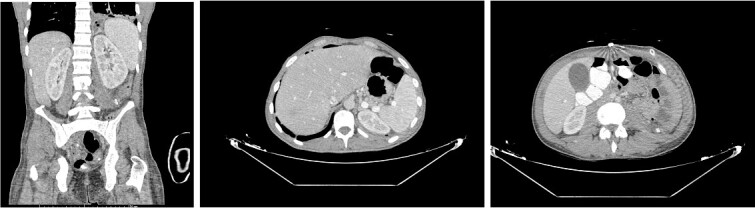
Postoperative CT done on the fourth day after surgery revealed a significant reduction in abcess size and improvement of the left lower lung lobe infiltration.
